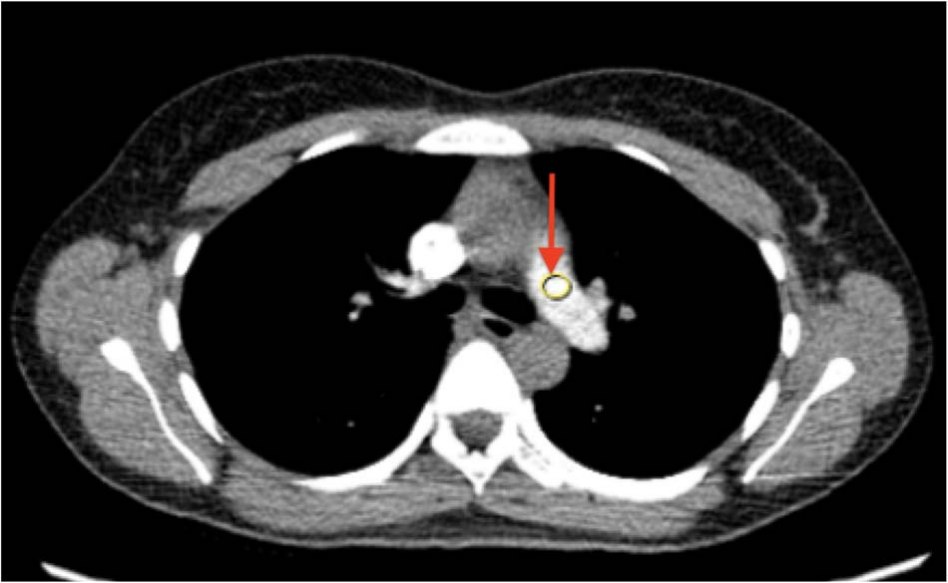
CT chest angiography confirming the absence of pulmonary embolism. Pulmonary arteries (arrow) indicate no evidence of acute pulmonary embolism. CT angiogram was performed following DVT diagnosis from ultrasound, and prior to mechanical thrombectomy.
